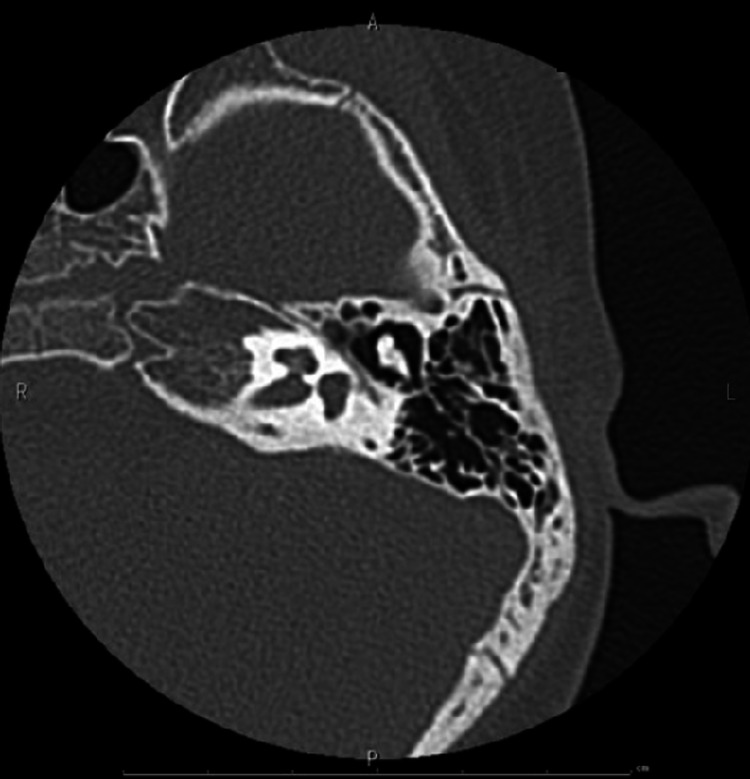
Imaging of Case 1: temporal bone CT (left) Healthy side. CT, computed tomography.
